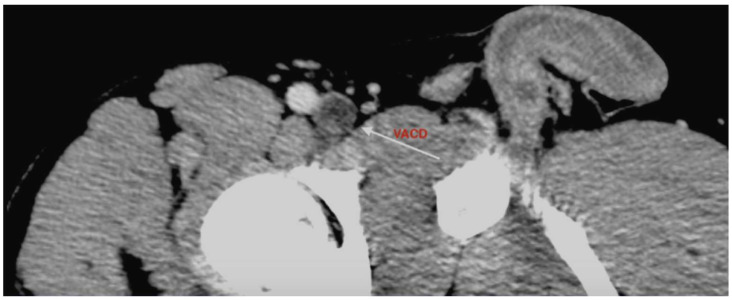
Cross-sectional CT angiography image demonstrating venous adventitial cystic disease; the arrow indicates the cystic lesion. The structure fills almost the entire lumen.
